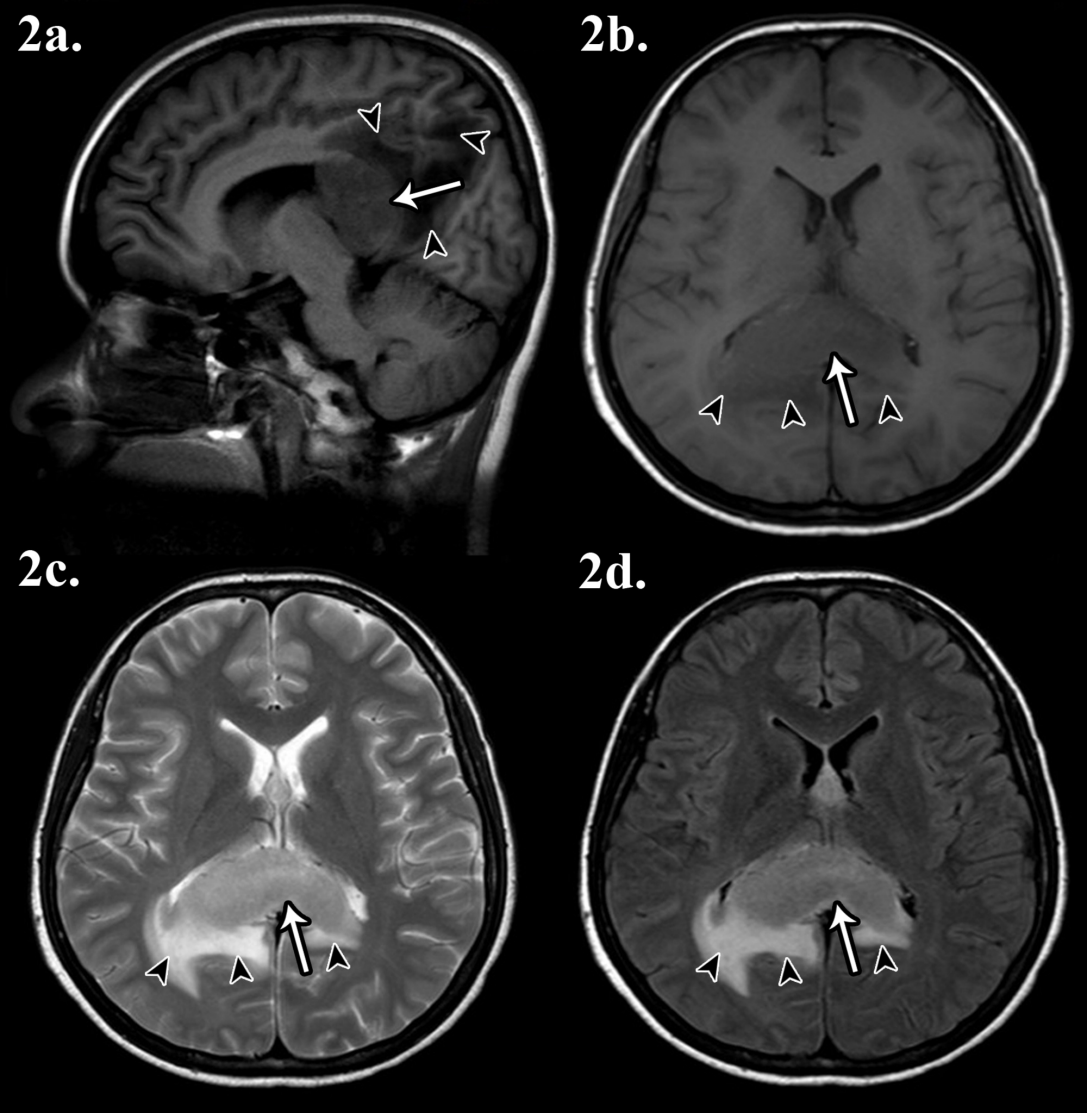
Magnetic resonance imaging (MRI) of the brain 2a) T1-weighted sagittal imaging; 2b) T1-weighted axial imaging; 2c) T2-weighted axial imaging; 2d) fluid attenuation inversion recovery (FLAIR) sequence imaging. A well-defined mass is visible in the splenium of corpus callosum (white arrow), crossing the midline. The mass is isointense on T1, hyperintense on T2, and hyperintense on FLAIR. There is associated white matter oedema (black arrowheads).
